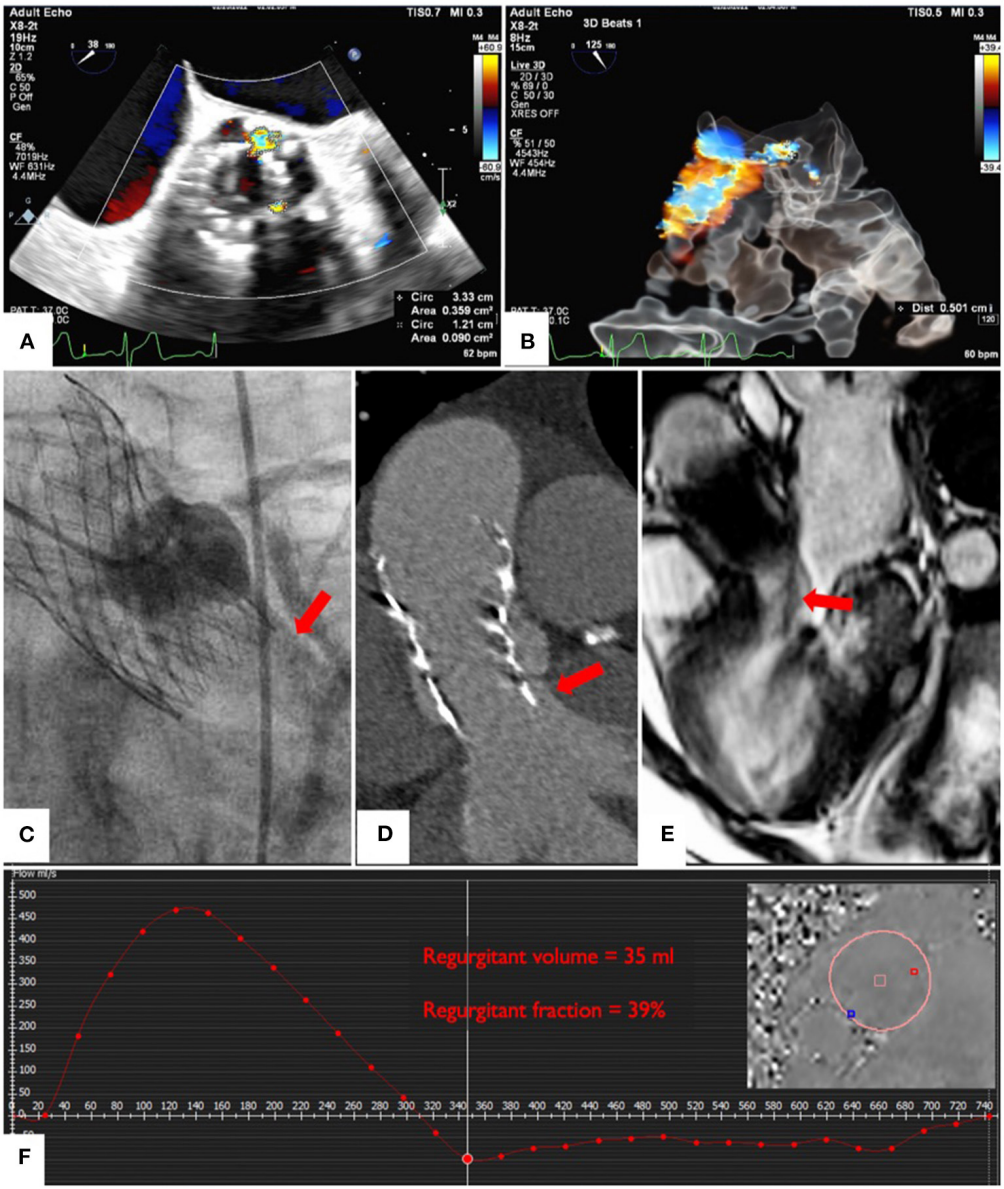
Multiparametric assessment of PVL severity. Echocardiographic quantification of jet size by planimetry (A) and by VC using glass image processing (B); (Supplementary Video 5). Angiographic assessment of PVL (C). Computed tomography showing prosthesis under-expansion (D). CMR of the largest PVL jet (E) and quantification of the total regurgitant volume and fraction (F); (Supplementary Video 6).
